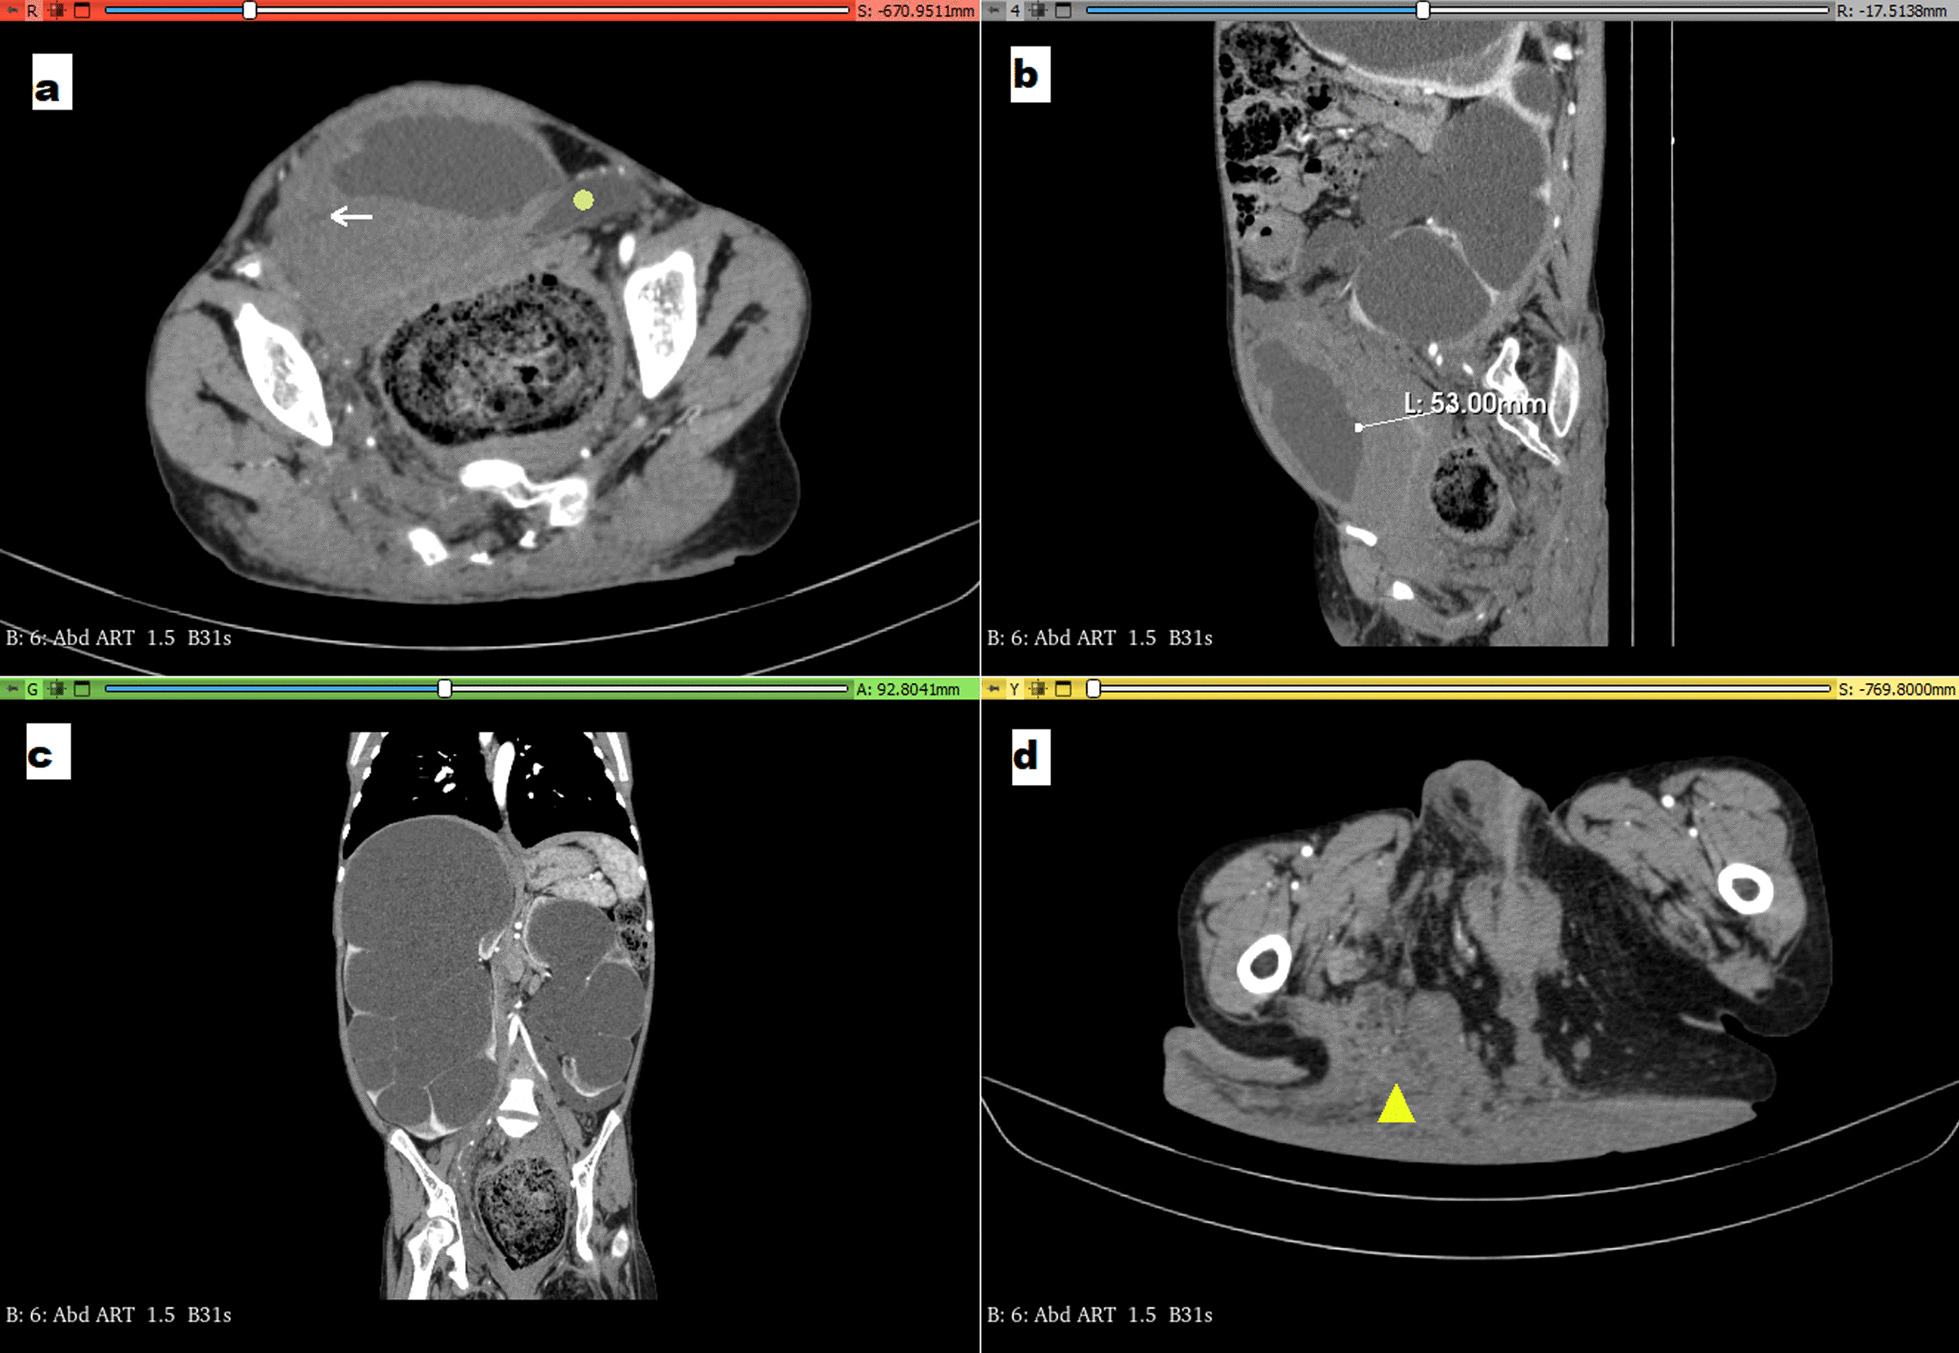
a Axial contrast-enhanced computed tomography (CT) image revealing the thickened wall of the urinary bladder with stenosis of the right ureter at the vesicoureteral junction (arrow) and the dilated left ureter (circle) just before joining the bladder b Sagittal CT image showing infiltration of the wall of the urinary bladder (53 mm thick) c Coronal CT image showing bilateral hydronephrosis d Axial CT image revealing the infiltration of neurofibromatosis in the right and left gluteal regions with dermal–hypodermal invasion (triangle)
